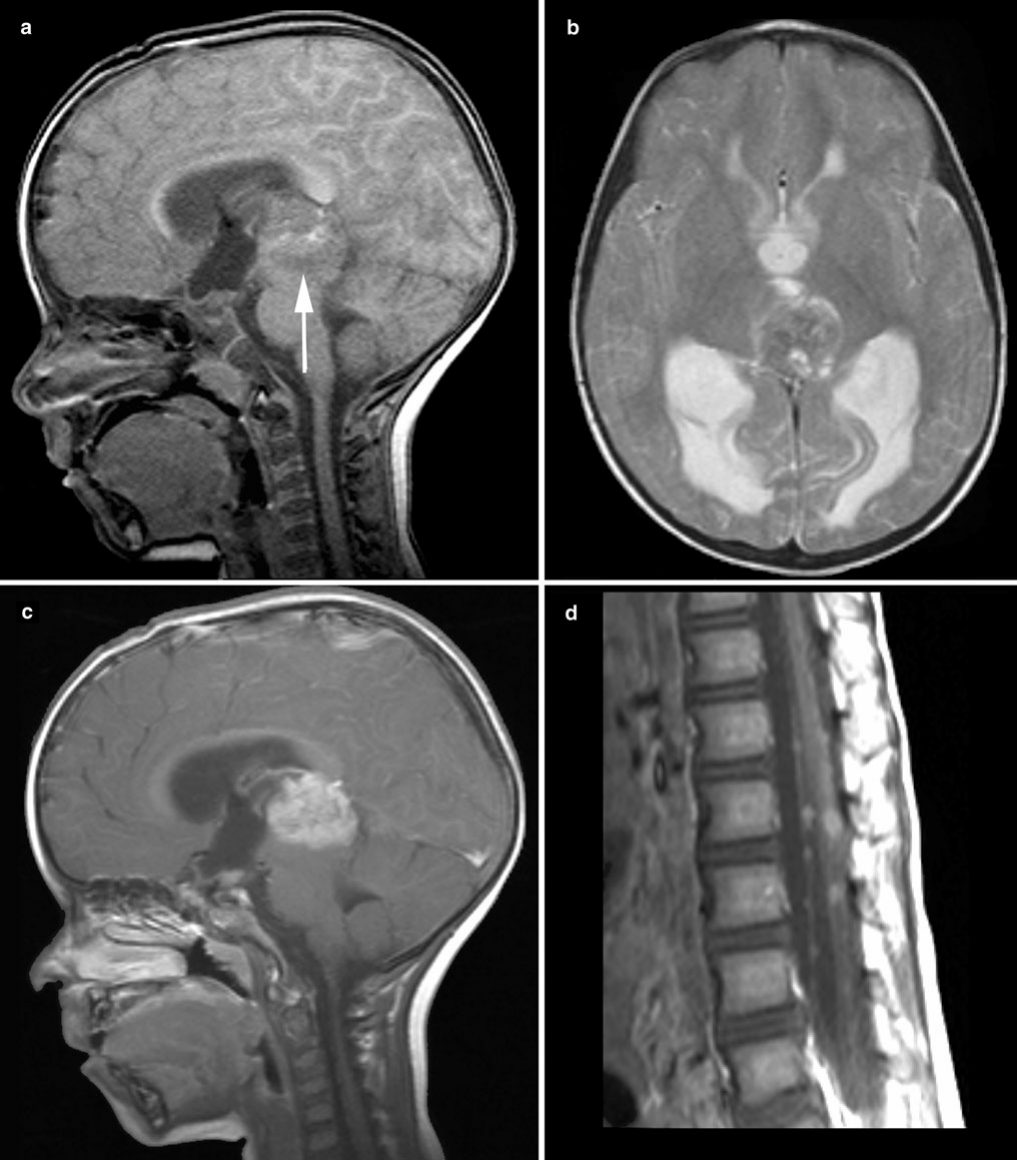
Solid pineoblastoma with hydrocephalus and extensive leptomeningeal metastases. Sagittal T1-weighted (a), axial T2-weighted (b), contrast-enhanced sagittal T1-weighted images of the brain (c) and spine (d) of patient 13. Pineoblastoma showed mostly isointense SI on both T1-weighted (a) and T2-weighted (b) MR images with respect to gray matter and homogenous contrast-enhancement (c). The large tumor mass (33 mm) showed compression on the brainstem (mesencephalon) and cerebral aquaduct (a, c) with secondary hydrocephalus (b). Multiple nodular leptomeningeal tumor seedings are present in the spinal canal (d)
